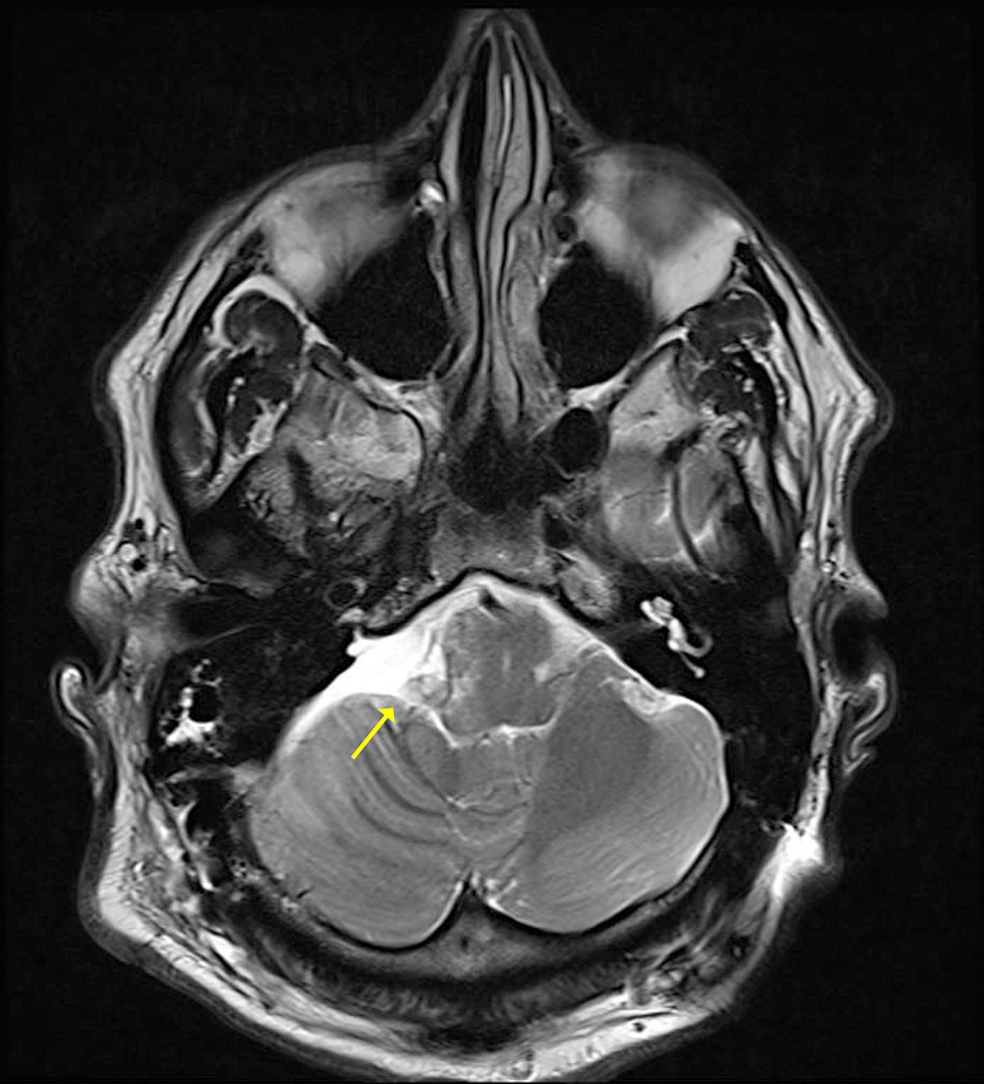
A postoperative T2-weighted image following a subtotal resection of the tumor shown by the arrow.
